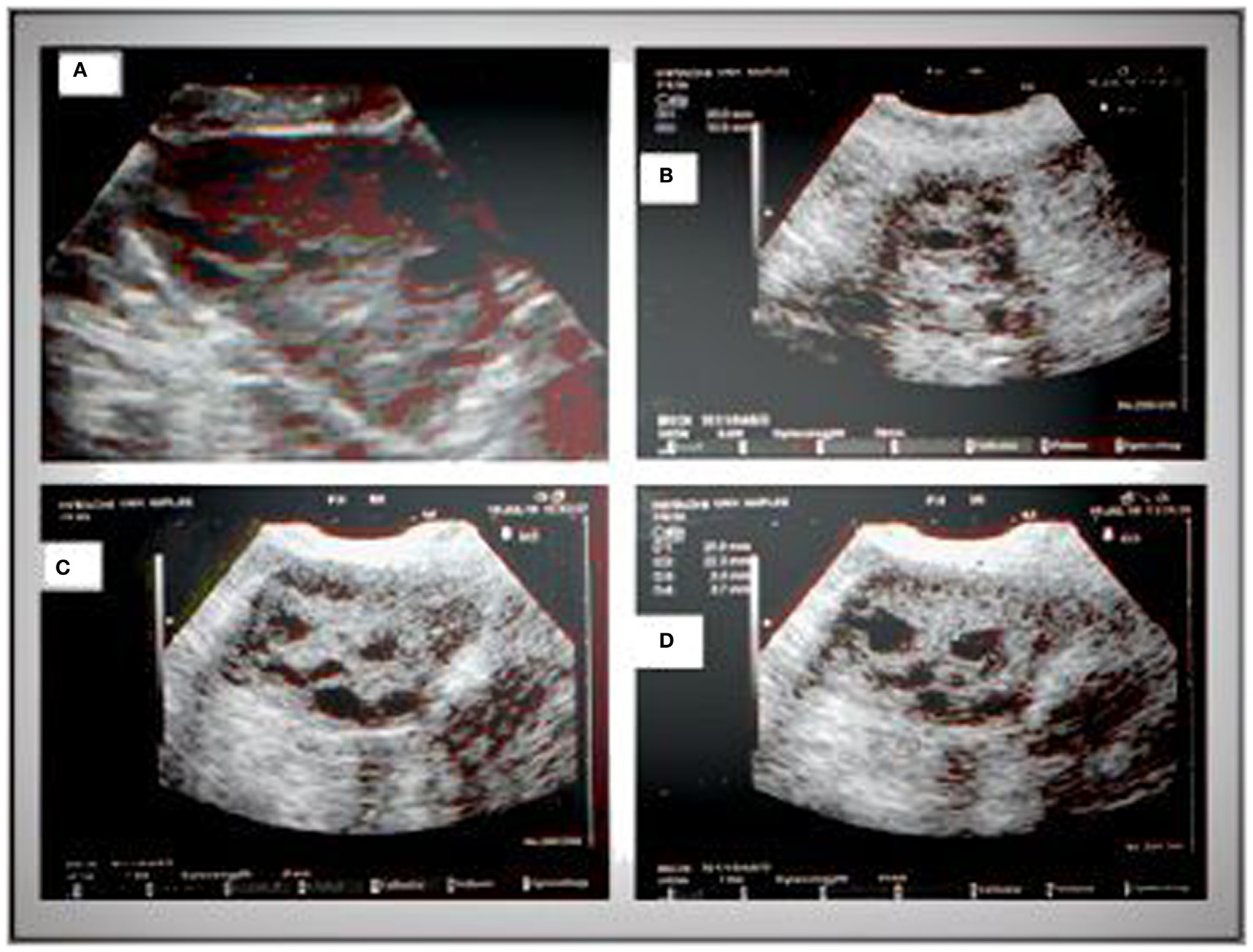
Sonographic pattern of a type B ovary (A). Note the ubiquitous arrangement of follicles and the absence of central echogenicity (B). Characteristic signs of the type B ovary are a more “globular” gonad versus a type A ovary (C), with attenuation of the typical dominance of longitudinal diameter and the presence of follicles with a mean diameter lower than those observed in type A (D).
